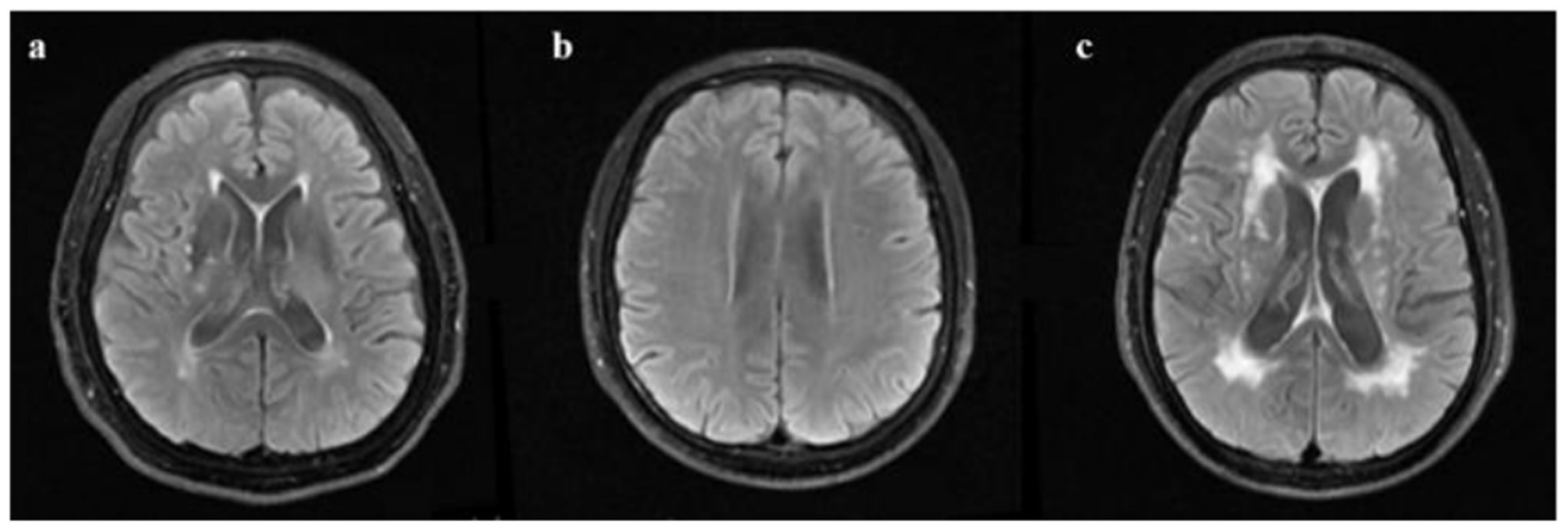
Fazekas scale criteria of periventricular white matter hyperintensities. (a) “Caps” or pencil-thin lining; (b) smooth “halo”; (c) irregular periventricular hyperintensity extending into the deep white matter (examples of T2 FLAIR from patients with aCSVD).
